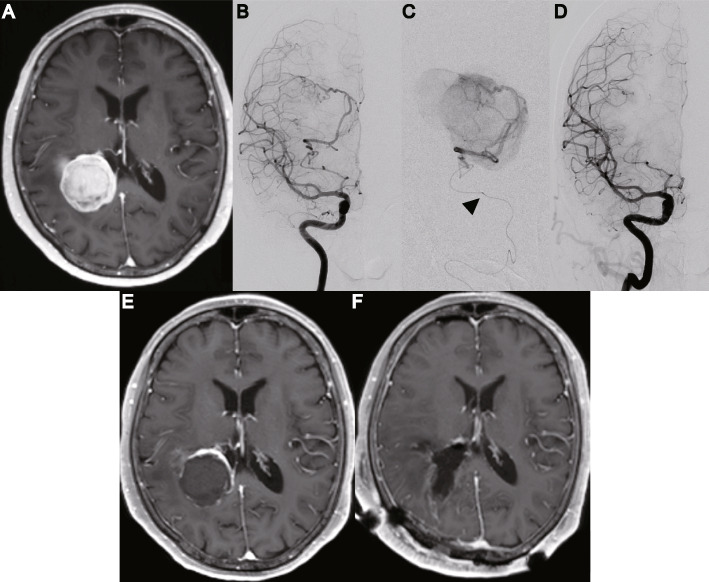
In Patient 2, a right lateral ventricular tumor was incidentally detected. a Preoperative magnetic resonance imaging (MRI) with gadolinium contrast showing a tumor in the right lateral ventricle (maximum diameter: 5.9 cm, tumor volume: 38.3 cm^3^). b Preoperative digital subtraction angiography (antero-posterior projection) reveals feeders originating solely from the anterior choroidal artery. The diameter of the AChA was 1.8 mm. c For endovascular treatment, a microcatheter is advanced beyond the angiographic plexal point (arrowhead). After a provocative test was performed using lidocaine and a barbiturate agent, the feeding arteries were obstructed using 100–300 µm trisacryl gelatin microspheres and coils. d Digital subtraction angiography (antero-posterior projection) reveals that the feeders projecting from the anterior choroidal artery have diminished after endovascular treatment. e After endovascular treatment, MRI shows a 97.9 % decrease of gadolinium-enhanced lesions. f After tumor removal via an intraparietal sulcus approach, MRI shows no residual tumor
